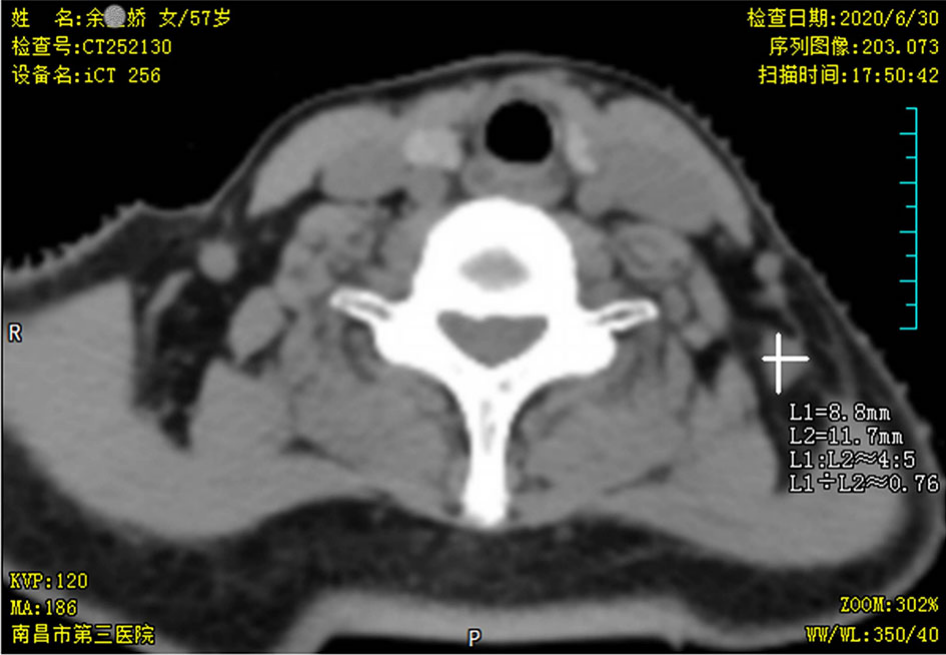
Neck computed tomography (CT) scan findings. Pretreatment axial neck computed tomography (CT) slice taken in the region of supraclavicular fossa revealed a 1.2 * 0.9-cm soft tissue mass in nodal level V.
